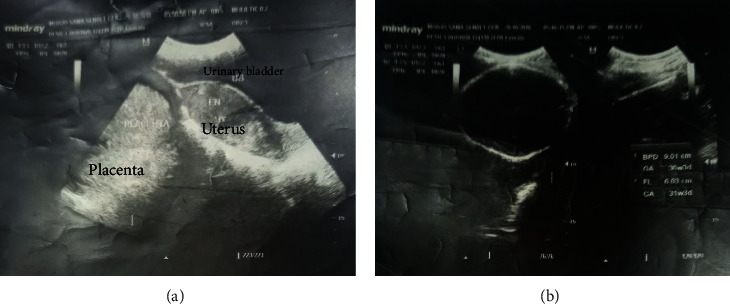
(a and b) Ultrasound findings of abdominal ectopic pregnancy.
